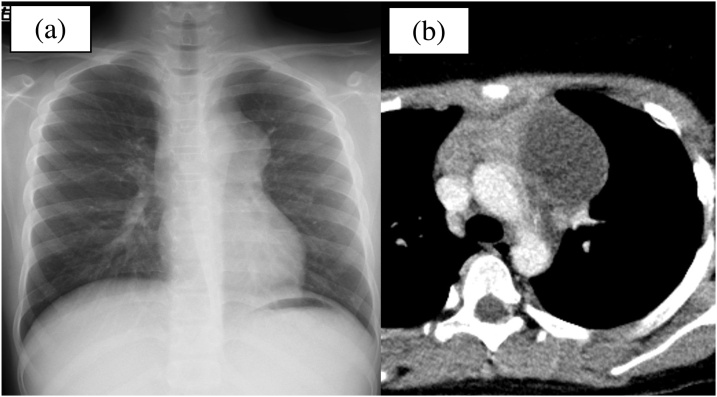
Patient images on the day of admission. (a) Chest radiography showing a large mediastinal mass. (b) Computed tomography showing a cystic mass with solid components in the anterior mediastinum.
